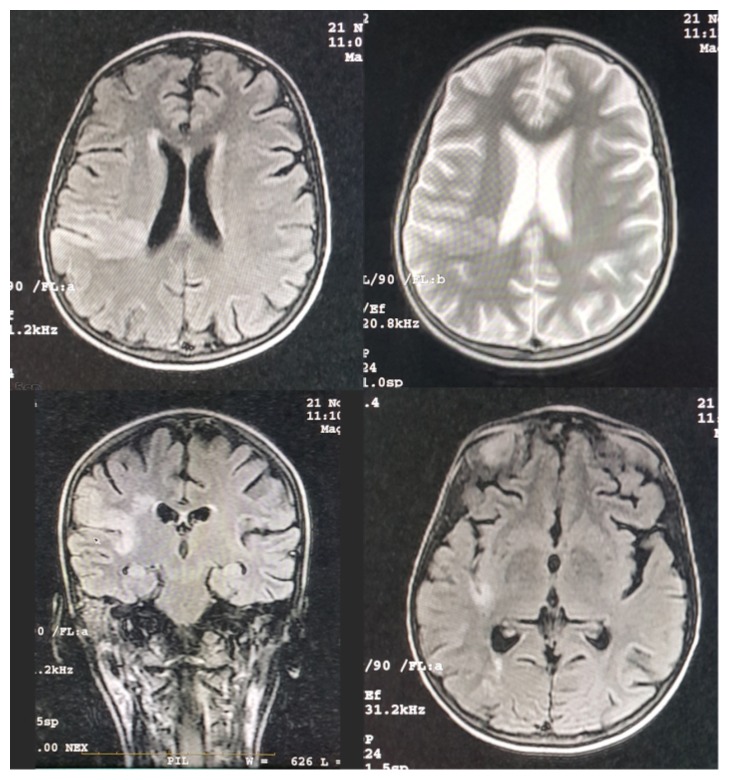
Patient MRI showing cortical and subcortical areas in the right temporoparietal fossa with high signal on T2 and FALIR studies, in addition to isointense foci in the cortical and subcortical area in the posterior part of the parietal lobe extending deeply through the posterior horn of the right lateral ventricle on T1 study.
